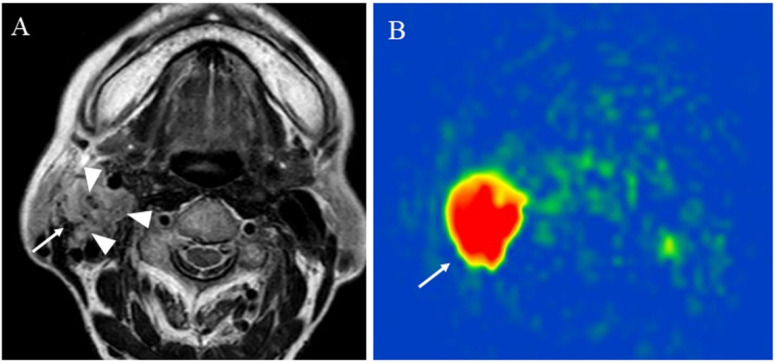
An 83-year-old female with the right carotid body paraganglioma: (A) T2-weighted image shows a right cervical mass (arrow) at the carotid artery bifurcation with high signal intensity and flow voids or salt and pepper appearance within the mass (arrowhead); (B) pCASL image shows abundant blood flow within the lesion (arrow) (mean of 303.04 mL/100 g/min).
